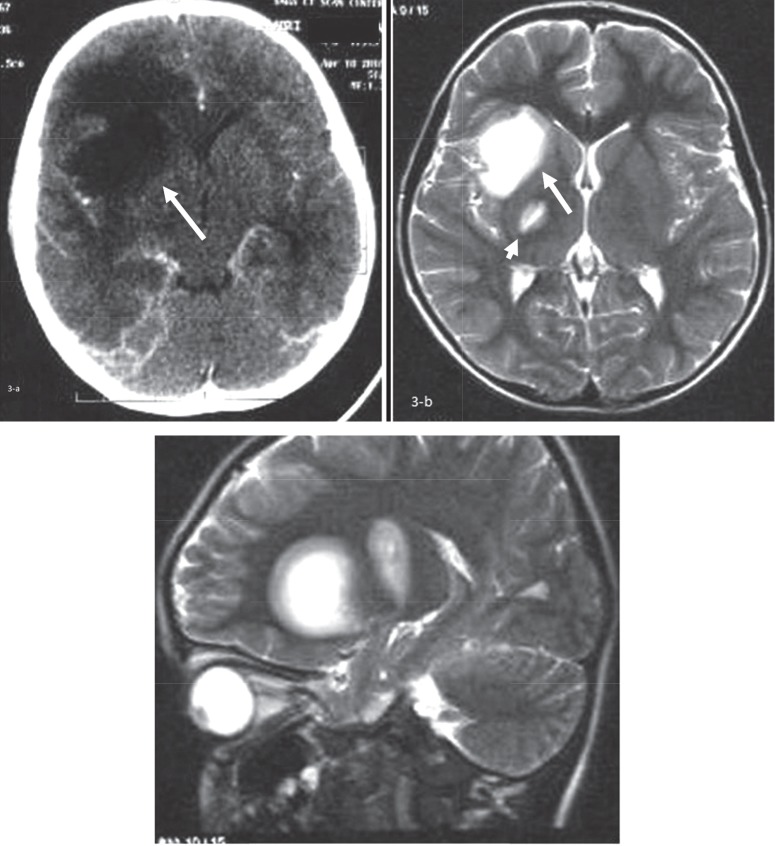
Brain CT scan with contrast demonstrated low attenuated subcortical areas in the right frontal and right lentiform nucleus without significant mass effect or post contrast enhancement (a-White arrow). The first brain MRI of the patient showed a couple of hypersignal lesions in T2-WI. The larger one is located in the right lentiform nucleus and insular subcortical white matter with surrounding halo (Large white arrow-b). The smaller one was located in the posterior limb of right internal capsule (Small white arrow-c
